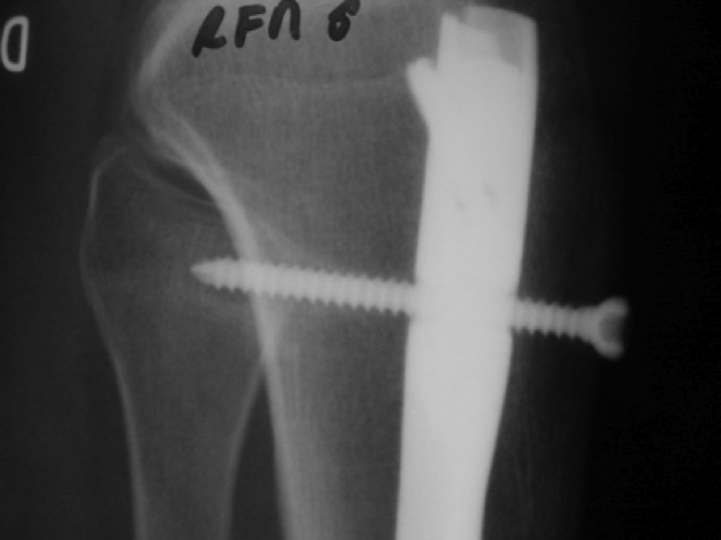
X-ray in lateral view with the oblique screw crossing the proximal tibiofibular joint.
